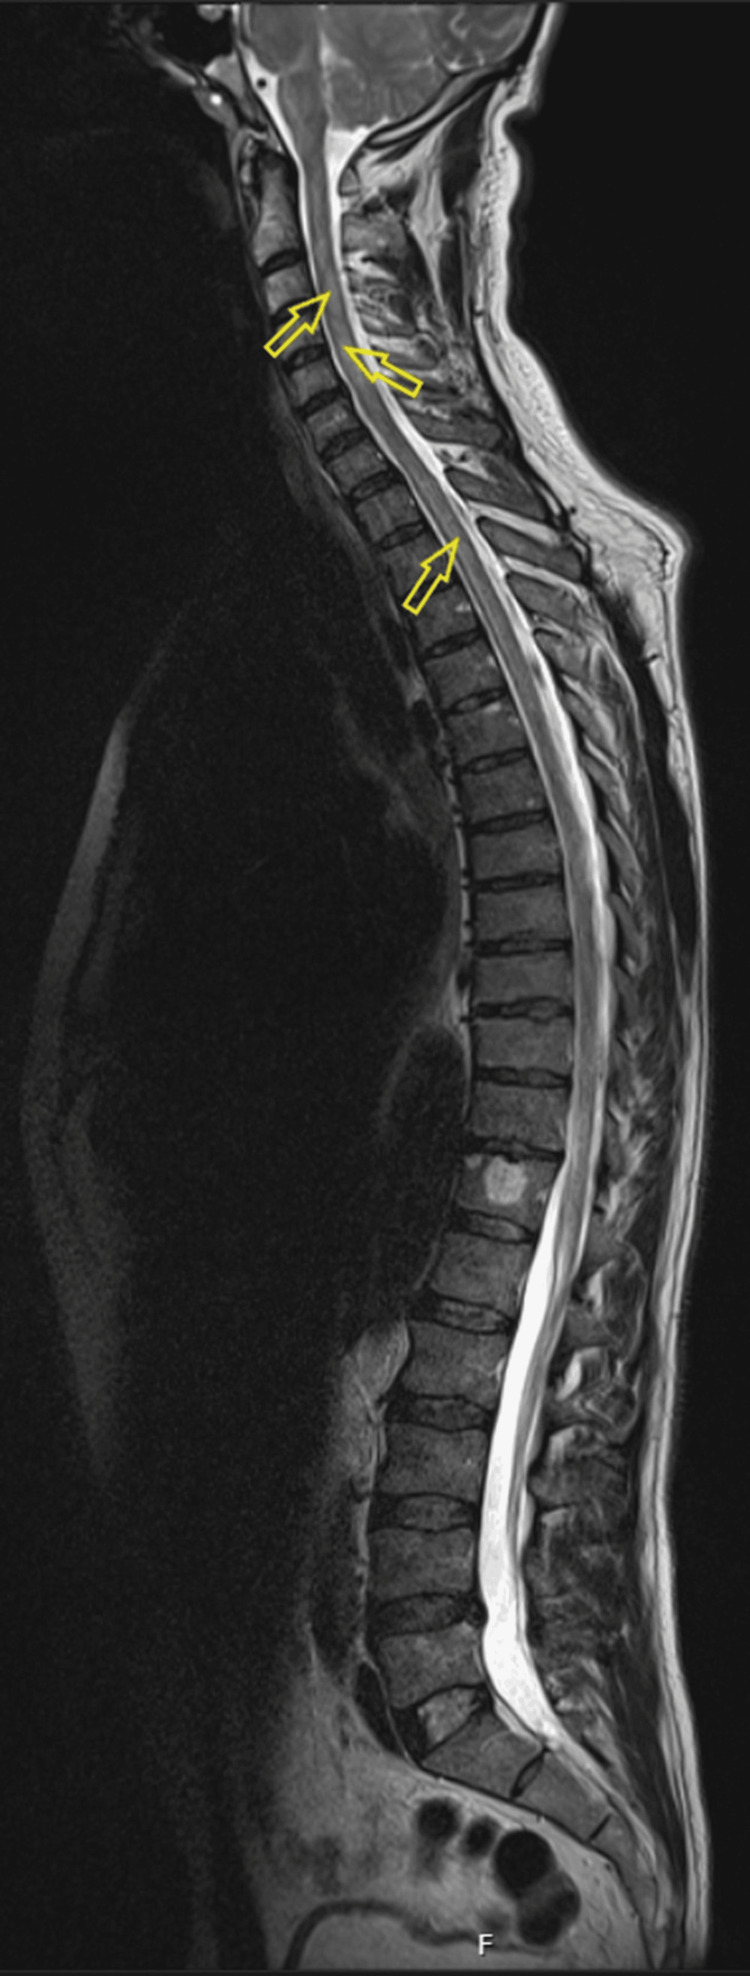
T2-weighted whole-spine MRI demonstrating multilevel short- and long-segment cervicothoracic hyperintense lesions consistent with longitudinally extensive transverse myelitis (LETM).
